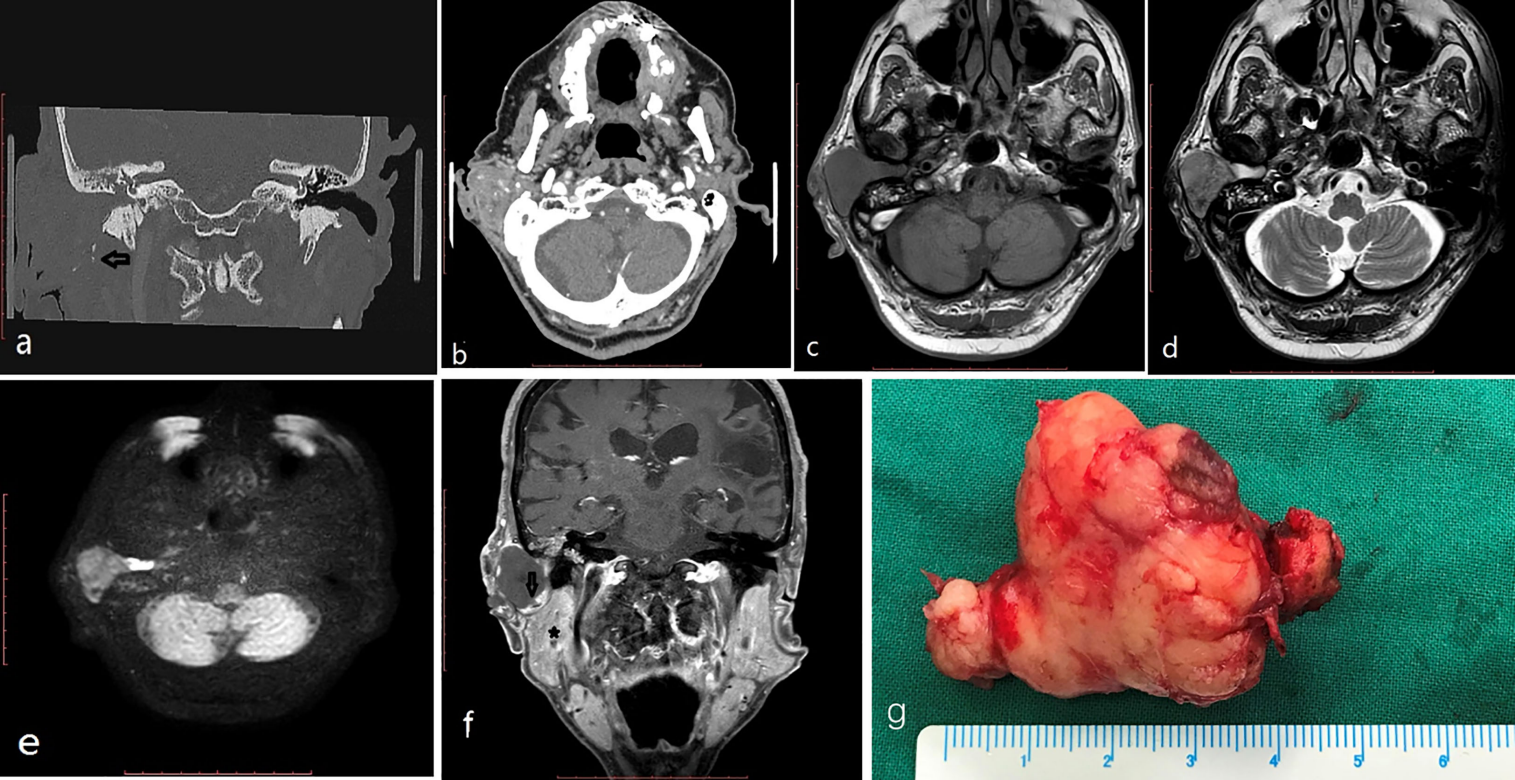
(case 8) An 84-year-old man had a 36mm max diameter ceruminous pleomorphic adenoma in the right EAC protruding out from the EAC and extending to the parotid (behind the mass, cholesteatoma was found in the EAC). CT: (A, B) calcified foci (black arrow) and heterogenous enhancement in the tumor. MRI (C-F):T1 low intensity (C), T2 intermediate and high signal intensity (D), DWI intermediate signal intensity (E) (behind the mass, cholesteatoma appeared as high intensity); the mass showed edge enhancement (black arrow) and was adjacent to the parotid (star) on coronal enhanced T1 (F). The mass is a lobulated solid mass on the gross appearance (G).
